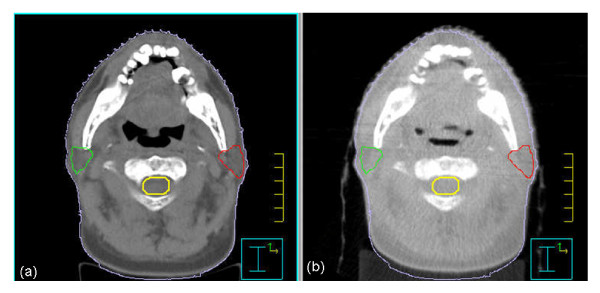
The transverse views of CT and the 2nd CBCT. The transverse views of the reference CT (a) and cone beam CT (b) after two weeks of the treatment. A slight change happened in the external contour and air cavity.
